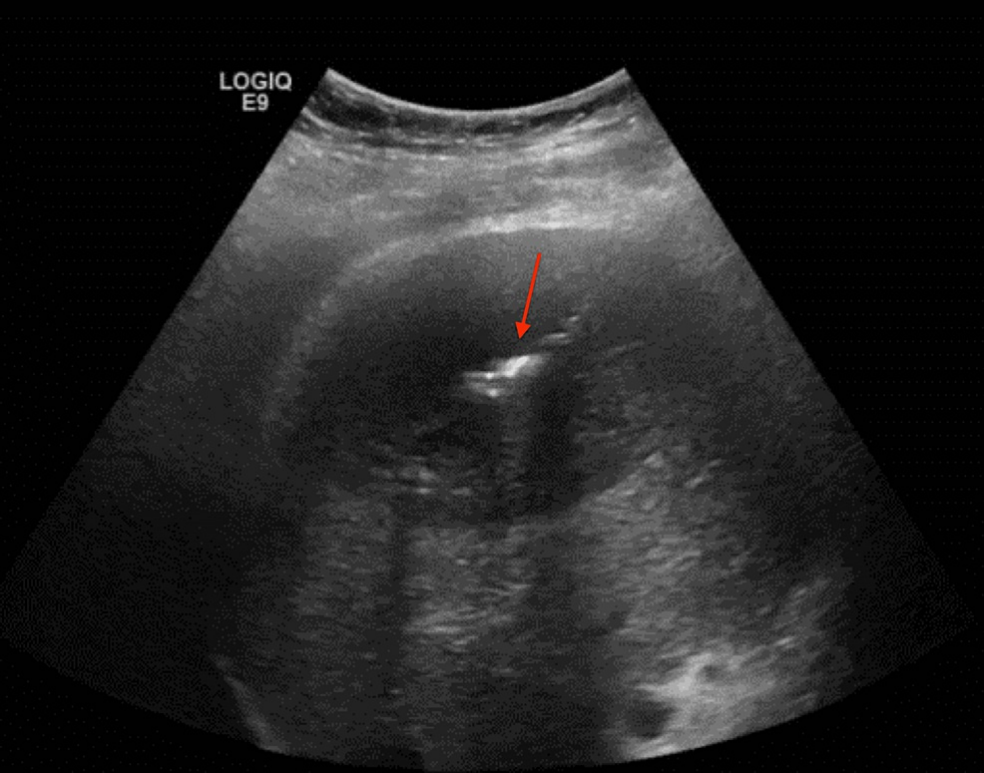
Ultrasound-guided needle aspiration and drain placement of hepatic abscess.
